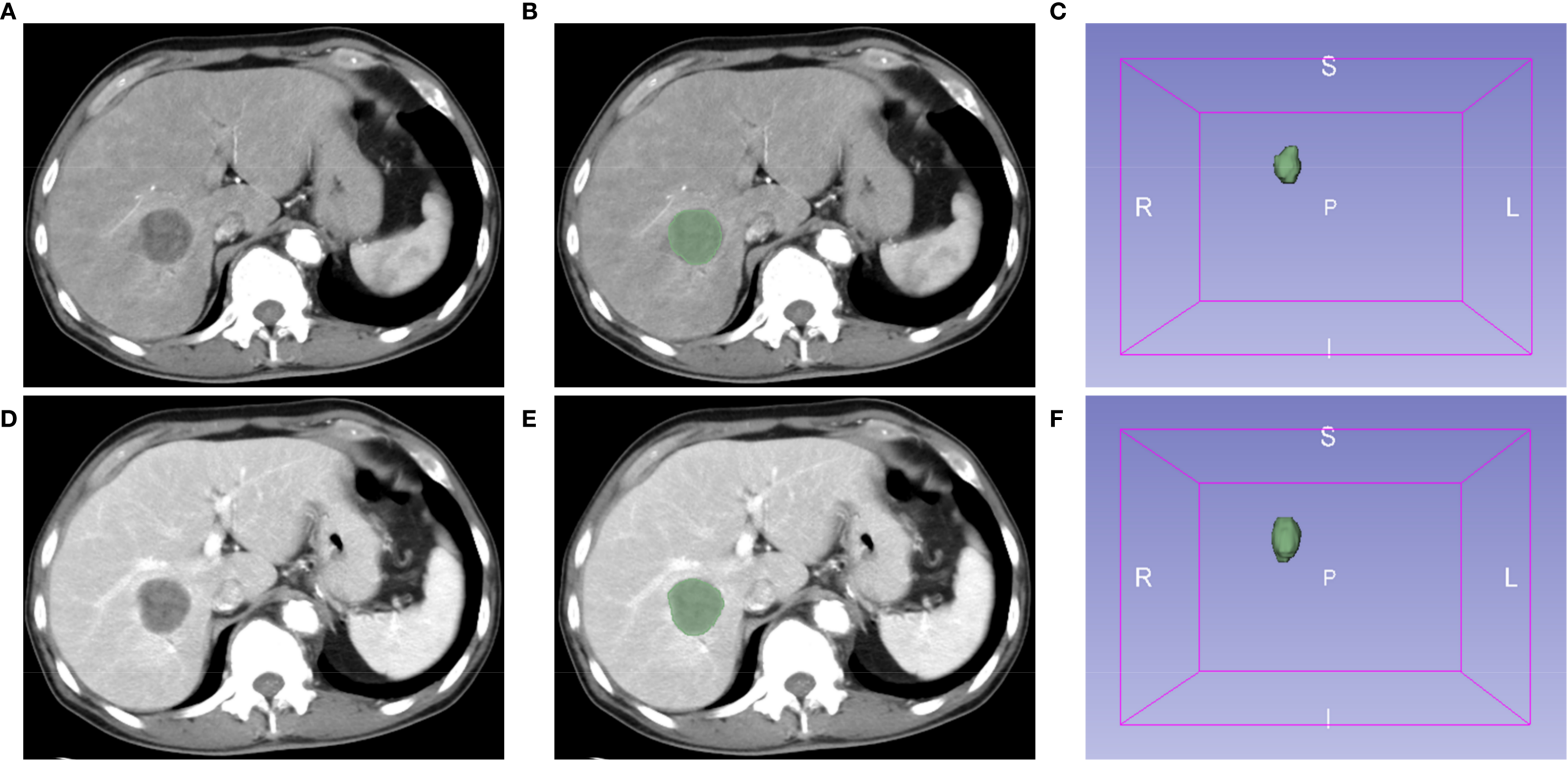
Extraction of radiomics features using the 3D Slicer software. Representative images of contrast-enhanced computed tomography (CT) arterial (A) and venous (D) phases. (B, E) Regions of interest (ROI). (C, F) The 3D reconstruction of tumors.
